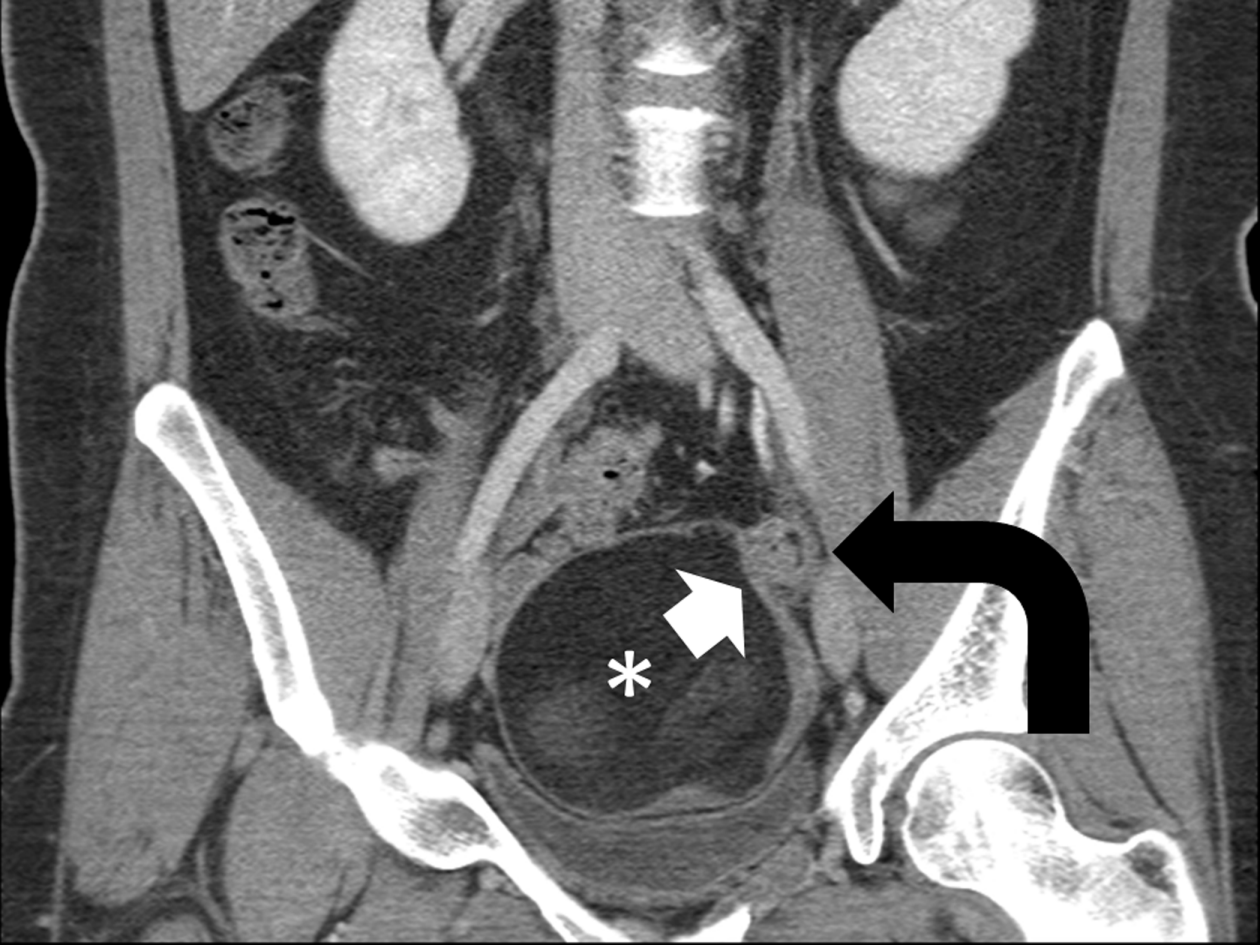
A 28-year-old woman with torsed left ovarian mature teratoma. Coronal contrast-enhanced CT scan shows a thickened pedicle (curved arrow) left superior to the fatty mass (asterisk). Denting (arrow) of the mass surface at the attachment site of the thickened pedicle is suggestive of a navel sign. Note that the thickened pedicle also shows a whirl sign.
